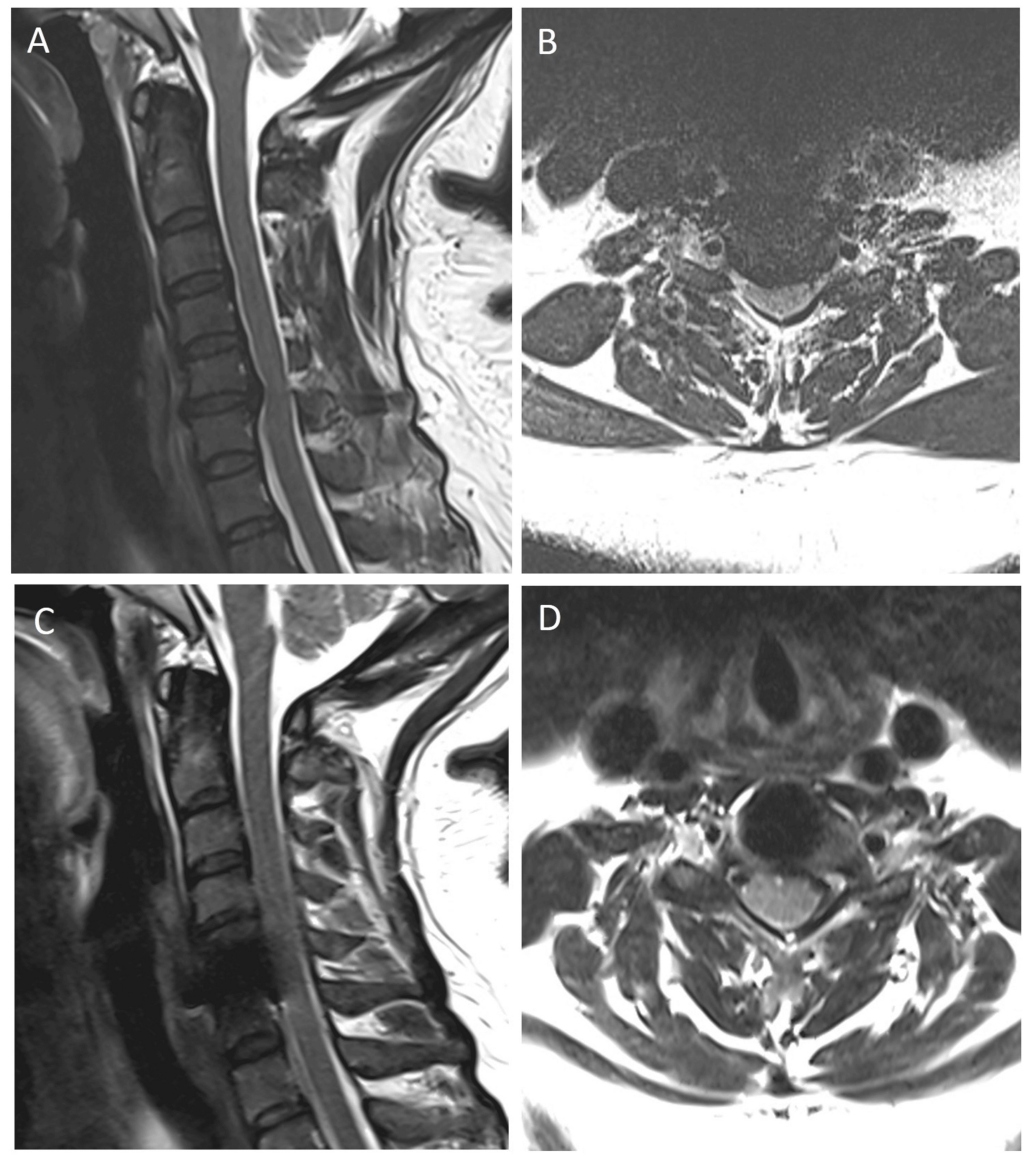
Pre- (A and B) and postoperative (C and D) T2-weighted MRI of the cervical spine Spinal cord compression with disc herniation over the C5–6 level was well decompressed. MRI: magnetic resonance imaging
